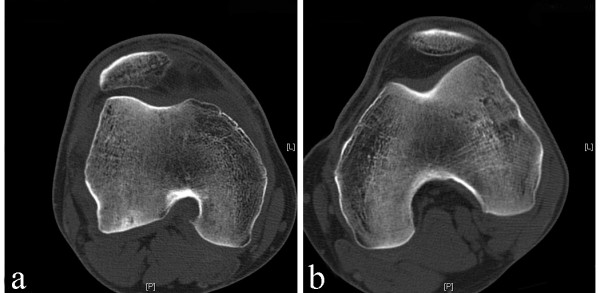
Computed tomography scans. Transversal layers of computed tomography scans of the affected (a) and contralateral (b) femur. Note the significant notch deformity in a.
